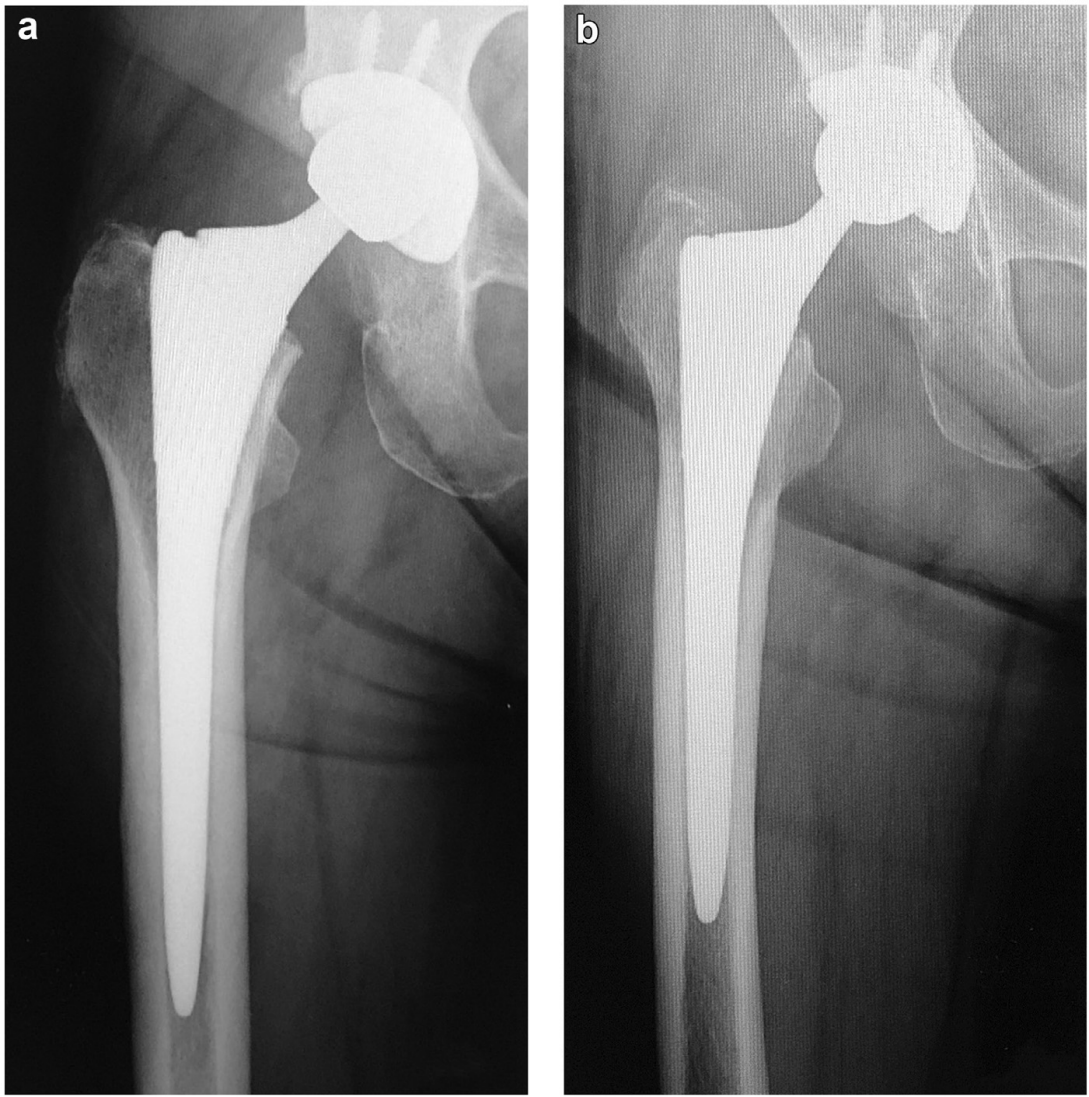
Radiographs of the (a) standard stem and (b) short stem (size 12).
